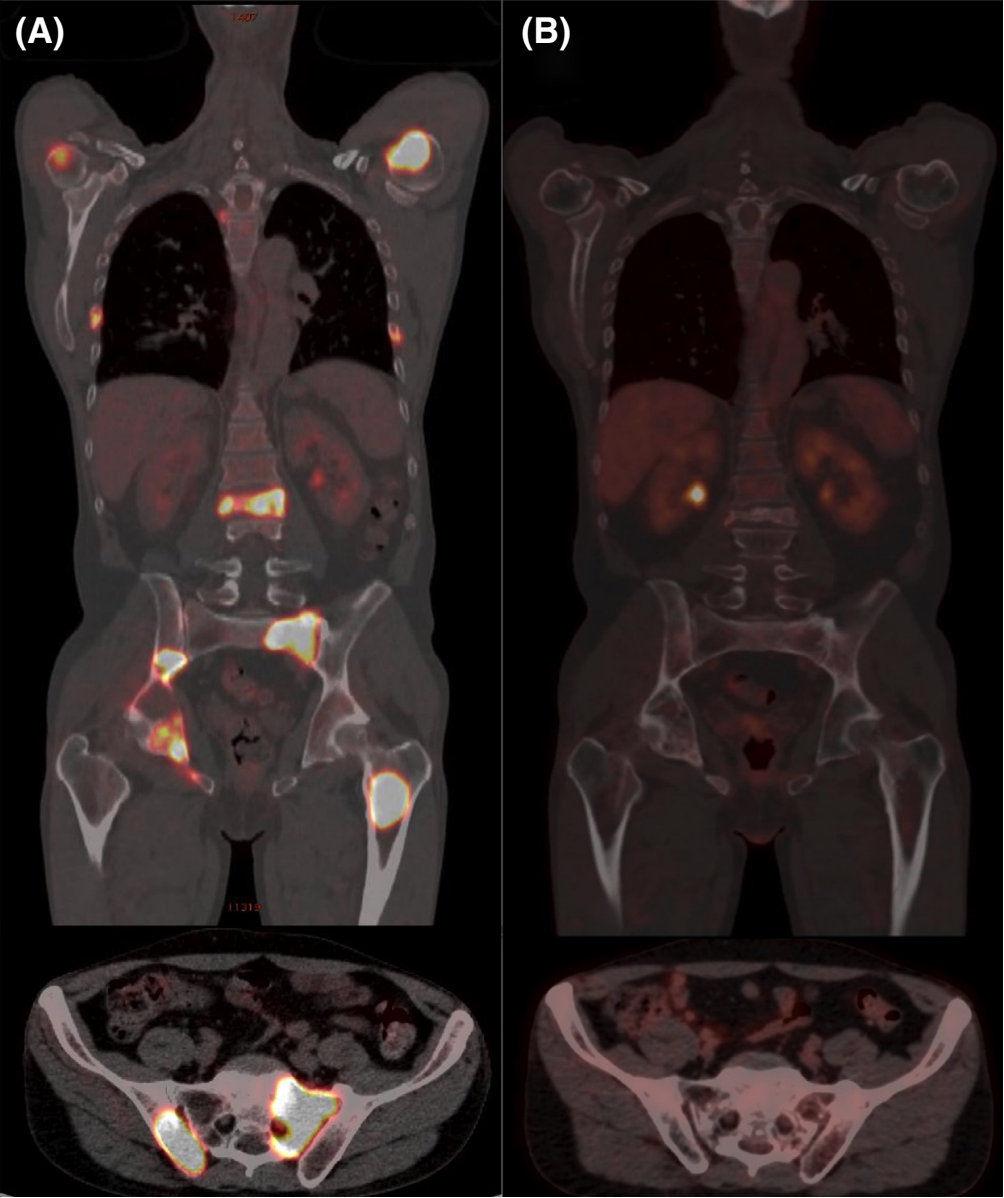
Axial and coronal fused FDG‐PET/CT images in the same patient before treatment (A) showed hypermetabolic bone lesions in both humeri, in some ribs, in a lumbar vertebra, in the pelvis (SUV max 34), and in the left femur. After treatment (B), the FDG uptake in the same areas was normal. Corresponding CT images depicted lytic bone lesions.
